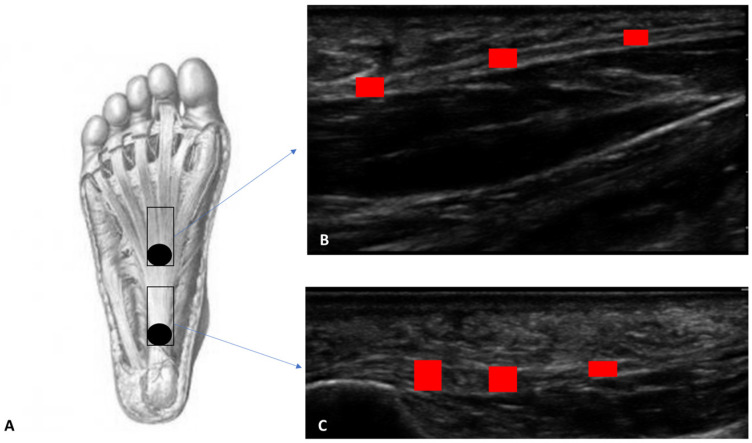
(A) Ultrasound measurement protocol of PF thickness at two levels of the plantar surface of the foot. The scans were taken on the long axis, paying close attention to maintaining the same structure in the center of the US monitoring image and keeping the probe perpendicular. (B) Level 1: at the calcaneal insertion of the PF up to 2 cm from it. (C) Level 2: in the middle third of the PF at 4–5 cm from the calcaneal insertion. Black circle: orientation of probe; red box: thickness of plantar fascia.
